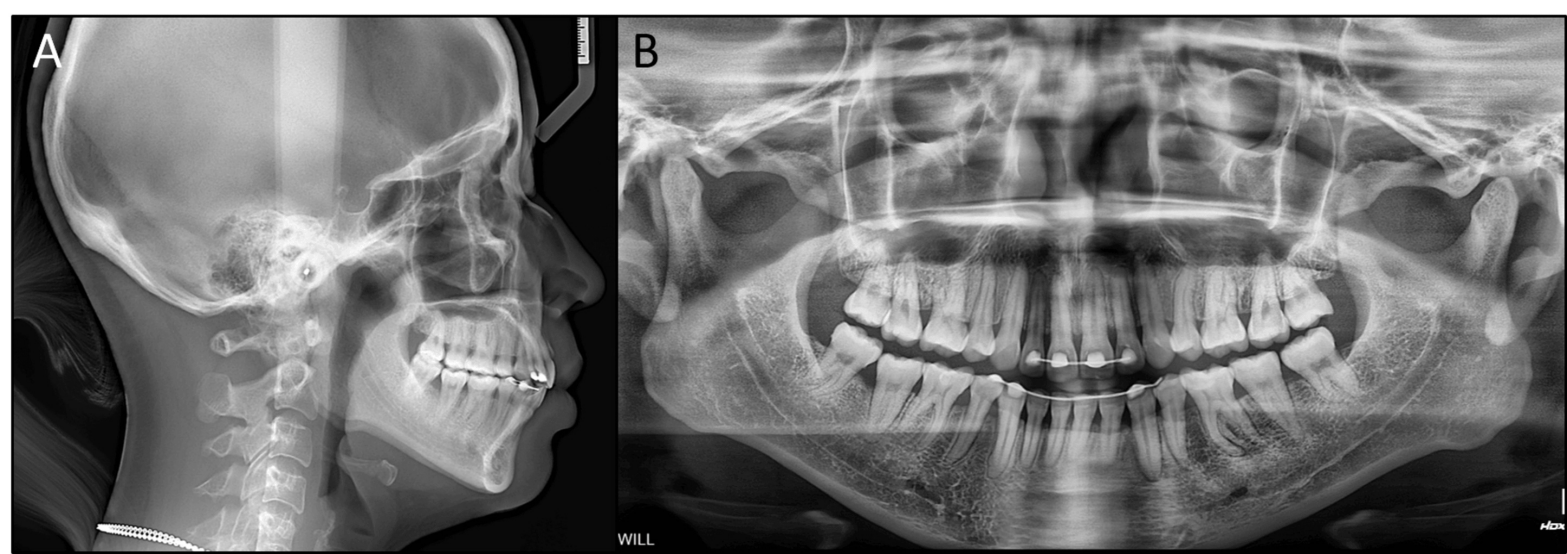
Post-treatment radiographs. (A) Lateral cephalometric radiograph, (B) panoramic radiograph.
